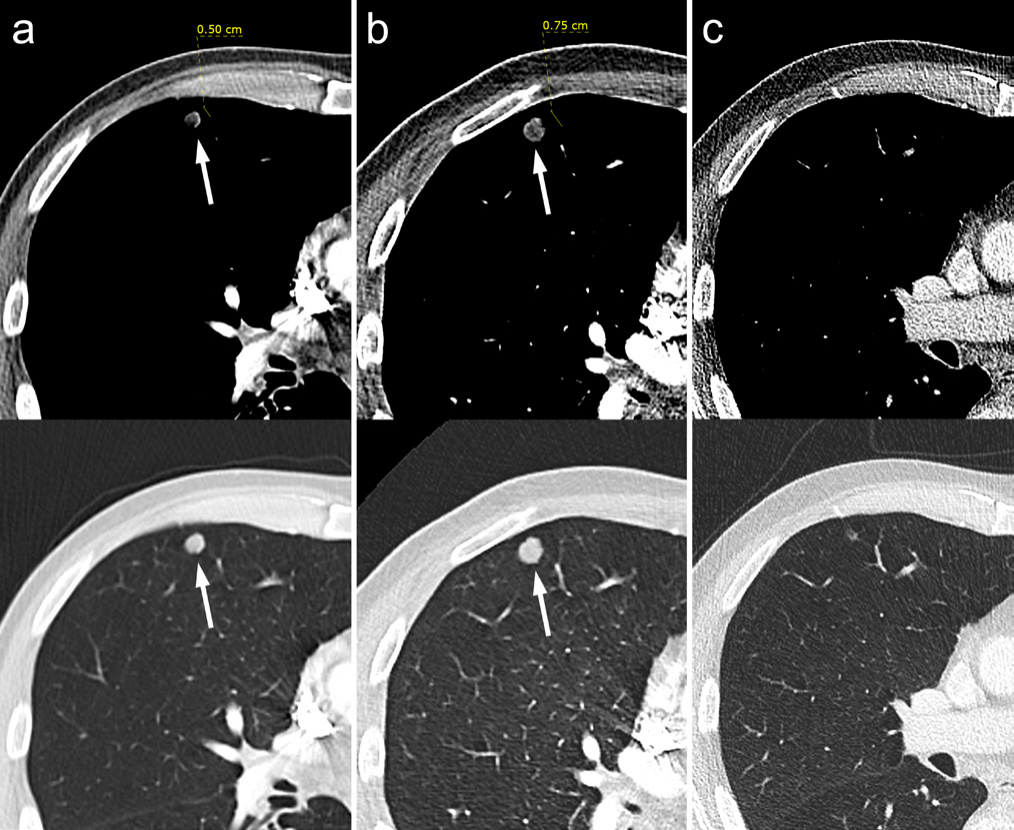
Axial CT images on soft-tissue and lung window settings demonstrating one of multiple low-density pulmonary nodules within the right lung on initial arterial-phase contrast-enhanced CT (A), which increases in size on a subsequent CTPA (b) and then can be seen to have almost completely resolved following chemotherapy treatment on subsequent restaging contrast-enhanced CT (C). CTPA, CT pulmonary angiography.
